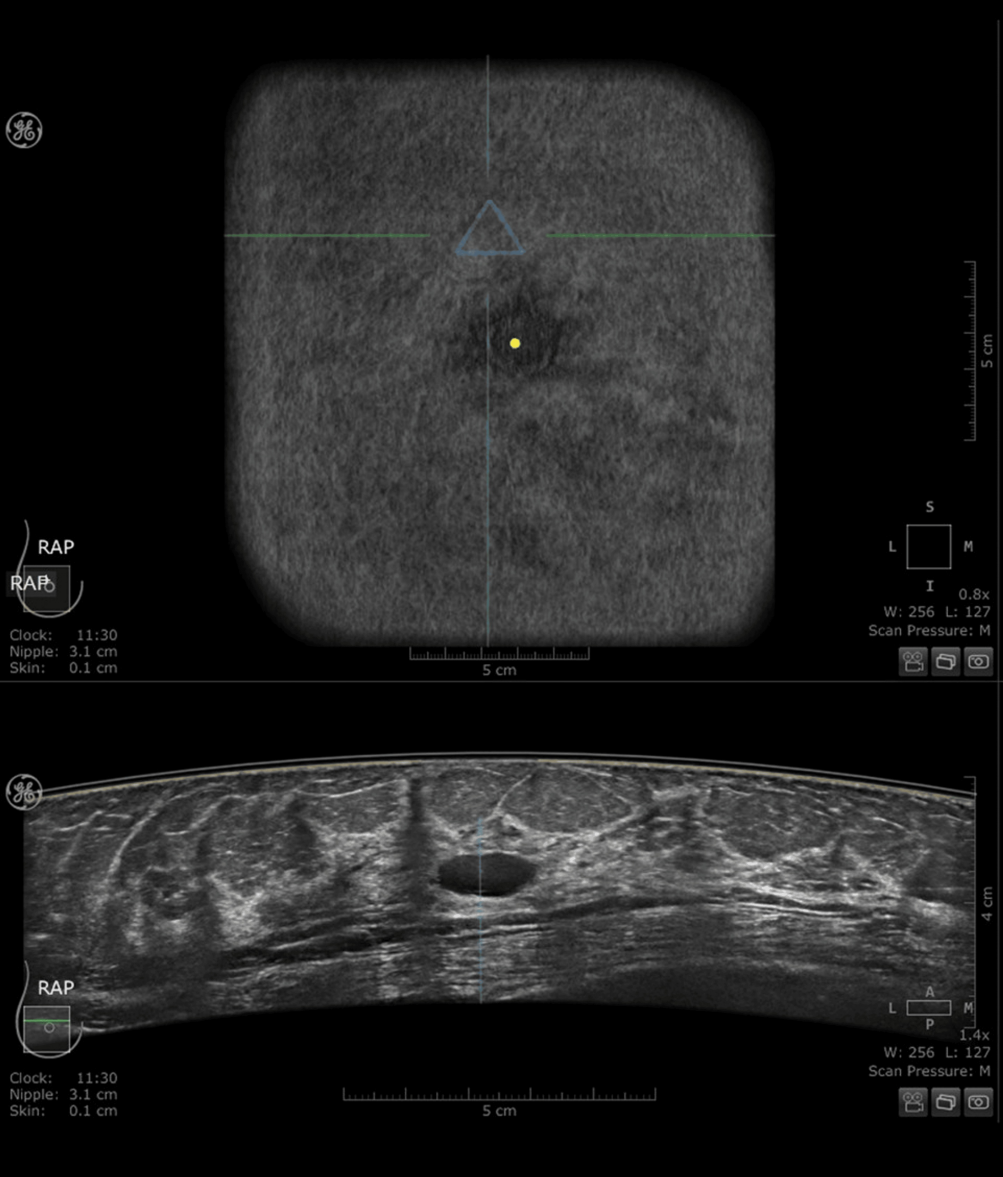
Representative ABUS workstation view demonstrating the digital tagging protocol for symptomatic patients. The top image (coronal plane) displays the dedicated "triangle" icon placed by the technologist to mark a palpable mass at the 11:30 clock position. The bottom image (transverse view) reveals the corresponding lesion directly aligned with the marker, facilitating correlation between the physical examination finding and the 3D volume review. 3D, three-dimensional; ABUS, automated breast ultrasound.
